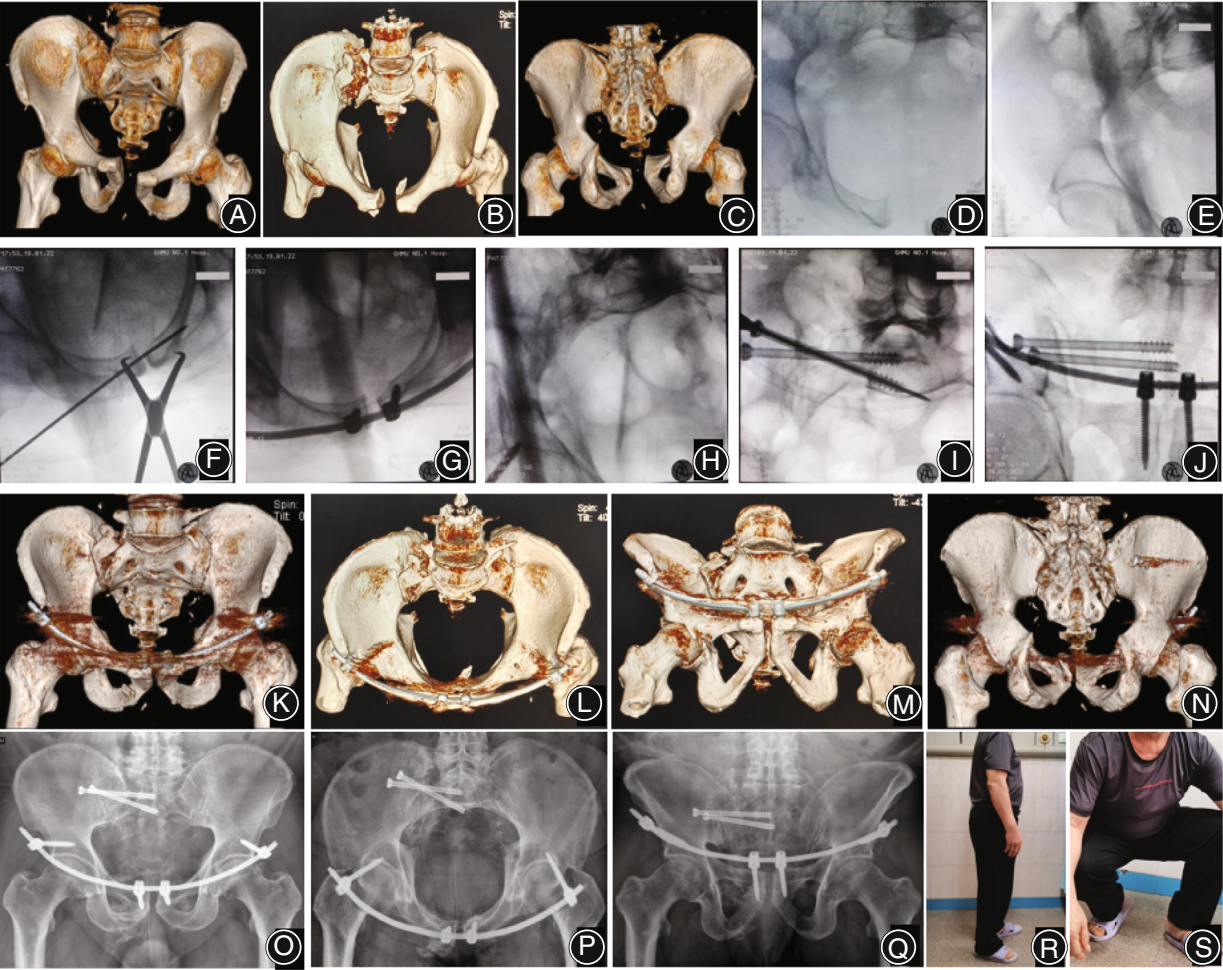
A 72‐year‐old man with pelvic ring injuries (Case 3) caused by falling from a height. (A, B, C) a preoperative three‐dimensional computed tomography image showing a vertical sacral fracture and dislocation of the symphysis pubis. (D, E, F, G, H, I, J) intraoperative imaging of fracture closed reduction and fixation. (K, L, M, N) two days postoperative three‐dimensional computed tomography image showing good reduction. (O, P, Q) plain radiograph image at six‐months postoperatively showing healing of the pelvic fracture and no loosening or fracture of the internal fixation. (R, S) follow‐up 1 year after surgery showing excellent function.
